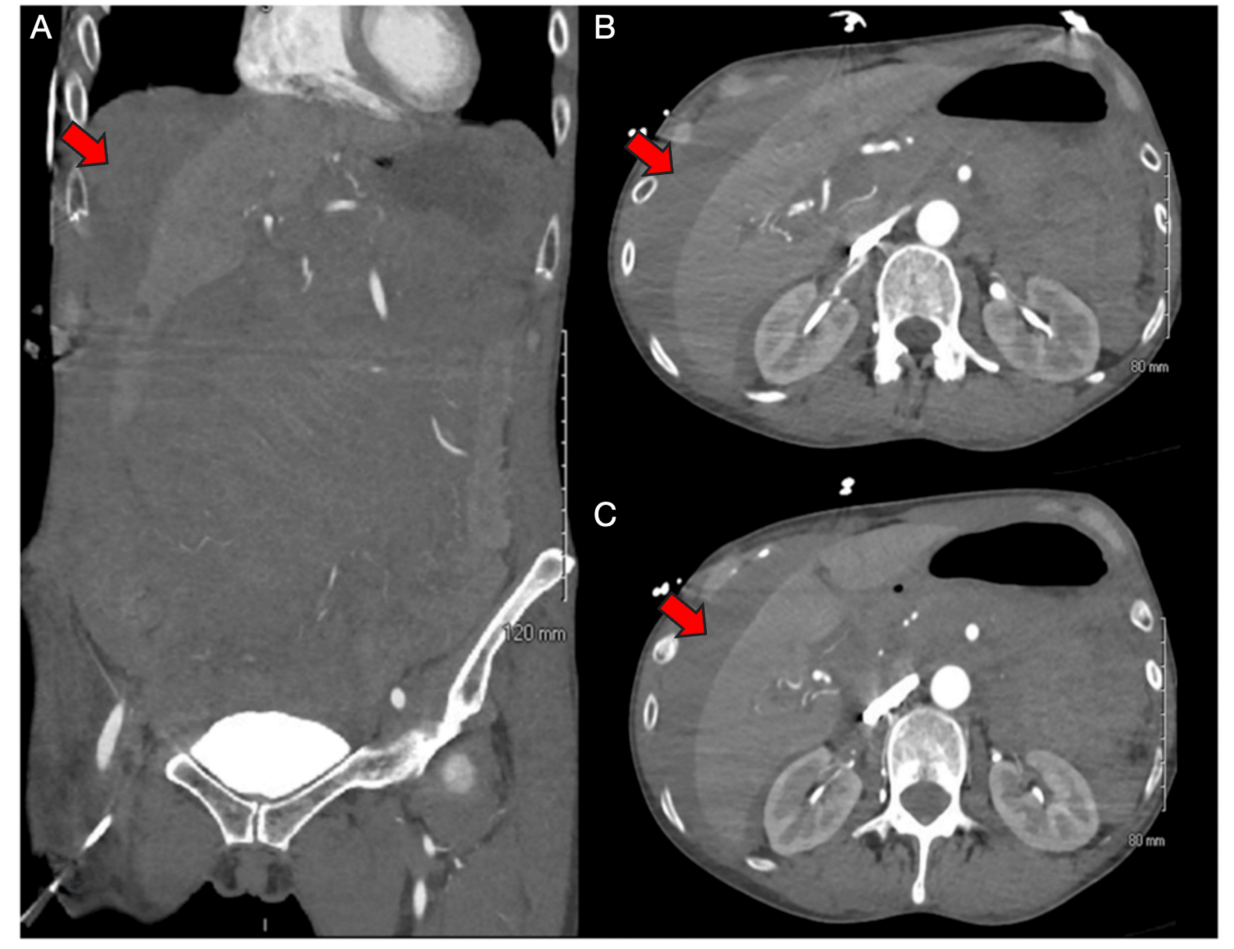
CTA of the abdomen and pelvis (two hours after the initial CT of the abdomen and pelvis). Repeat CTA of the abdomen and pelvis performed approximately two hours after the initial study shows no definite source of active contrast extravasation. The previously described small focus of contrast extravasation is less well delineated. Aorta and major abdominal vessels (celiac trunk, SMA, renal arteries) appear normal without stenosis. There is no evidence of aortic injury, GI bleeding, or new pathology. The IVC remains flattened, and the right femoral central line is unchanged. Overall, there is no interval change in the moderate hemoperitoneum, and no clear source of ongoing bleeding was identified (A) Coronal view: moderate hemoperitoneum (red arrow); no definite active contrast extravasation identified. (B) Axial view (superior): moderate hemoperitoneum (red arrow); no definite source of bleeding; abdominal vessels appear normal. (C) Axial view (inferior): stable hemoperitoneum (red arrow); no active extravasation or new pathology. CTA: computed tomography angiogram; CT: computed tomography; SMA: superior mesenteric artery; GI: gastrointestinal; IVC: inferior vena cava
